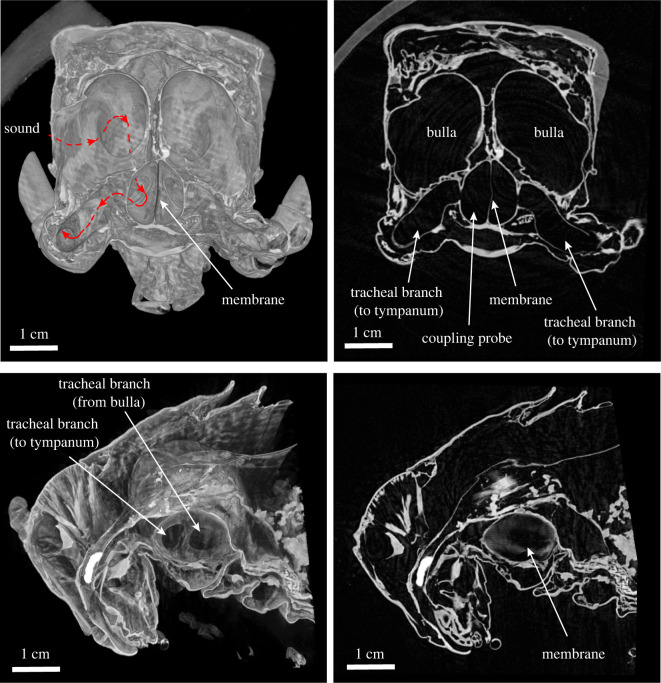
The *μ*-CT images of the membrane (septum) below the bulla, connecting the left and right trachea of a male *P. cieloi*. The red arrows show the route of the sound stimulus as it travels through the acoustic trachea.
